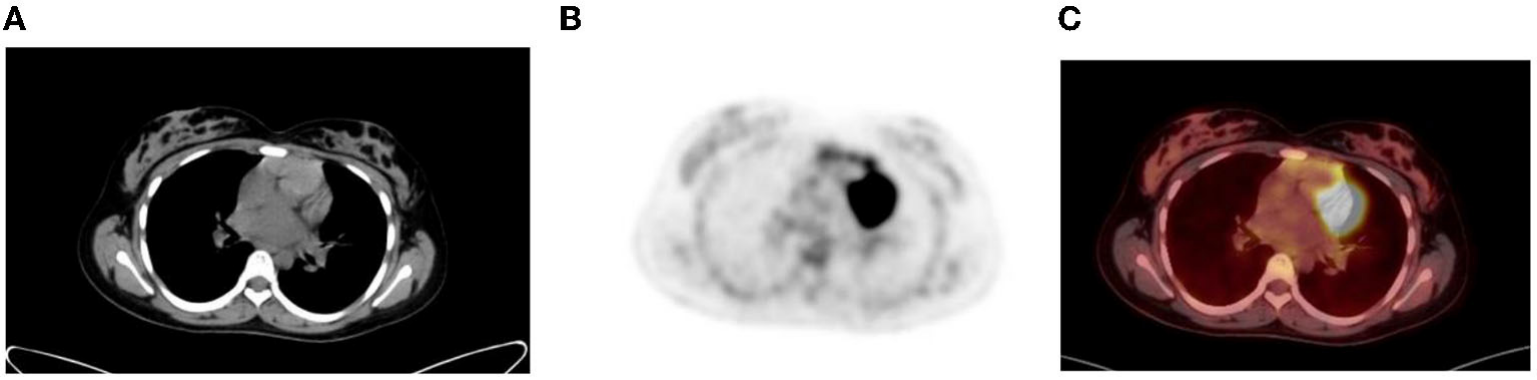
Diffuse uptake in the breast tissue in a 17-year-old female with embryonal rhabdomyosarcoma referred for interim PET study. CT (A) PET (B) and fused PET/CT (C) showing mild low grade uptake in bilateral breast tissue.
